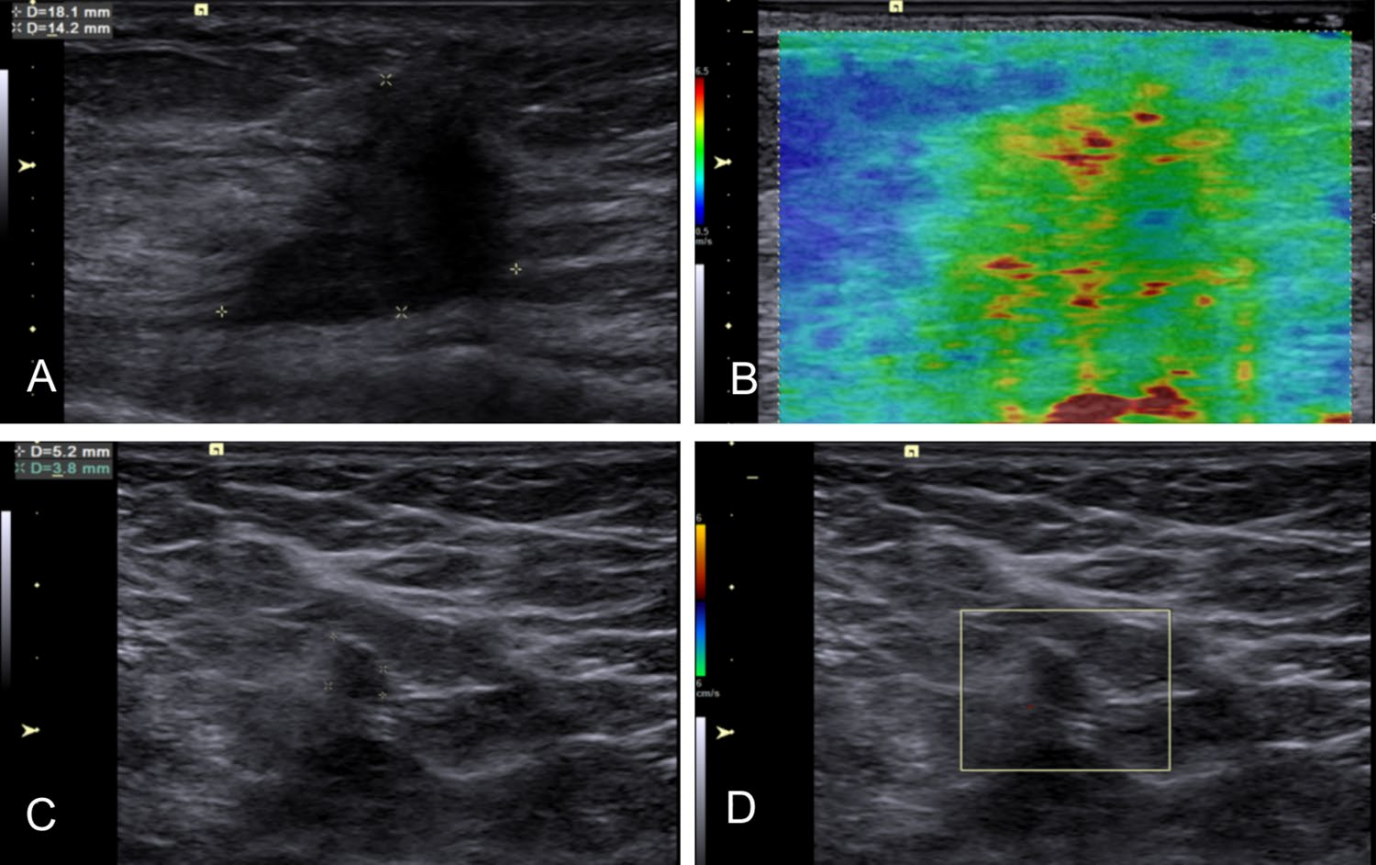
The characteristics of breast SWE and axilla US in a cases with positive axilla level III after NAT. A 53-year-old case with T2N3 Luminal-A breast cancer was found to have residual metastasis in 14 LNs in axilla level I, 3 in axilla level II, and 2 in axilla level III according to ALND result. A A solid, hypo-echogenic breast lesion measuring 18.1 × 4.2 mm was found on US. B The breast lesion showed a SWVmax value of 5.30 m/s on the SWE-velocity map. C–D Several suspicious small LNs were identified in axilla level I by using axilla US, characterized by ratio of long axis diameter to short axis diameter < 2, irregular shape, ill-defined margin, effacement of the fatty hilum, and absence of hilar blood flow. No LNs were detected in axilla level II or III on US. Based on the model combing breast SWVmax of 5.30 m/s and positive axilla level I on US, the predictive result indicated the presence of residual metastasis in axilla level III. SWE, shear wave elastography; US, ultrasound; NAT, neoadjuvant therapy; LN, lymph node; ALND, axillary lymph node dissection; SWV, shear wave velocity
